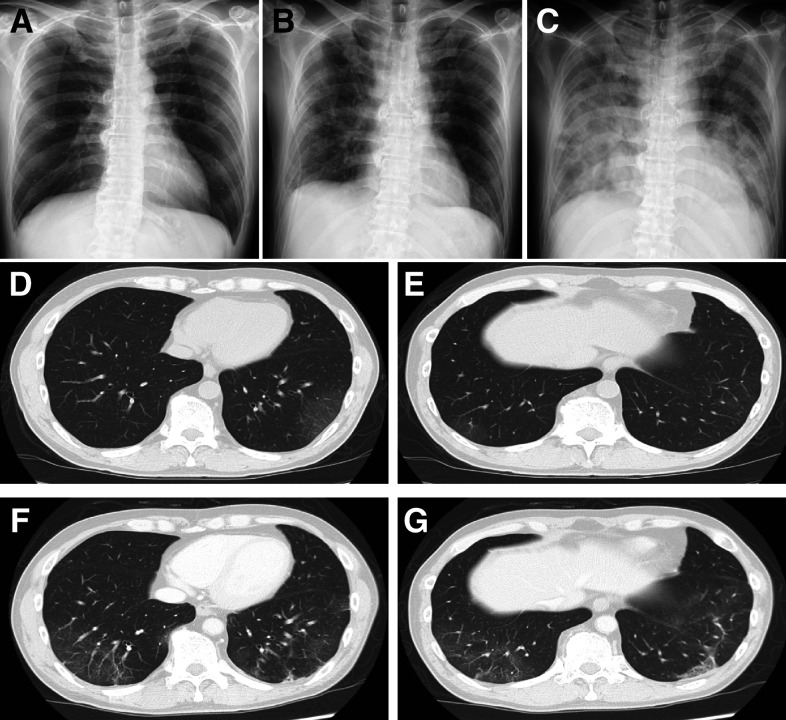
Two modalities of chest imaging studies in the COVID-19 patient. Chest X-rays. (A) March 31 (day 1); (B) April 27 (day 28); (C) May 4 (day 35). Chest computed tomography shows mild bilateral ground-glass opacities (GGOs) in the lower lobes (D and E: March 31, day 1). Part of the previous GGO disappeared, and a new subpleural GGO appeared (F and G: April 10, day 11).
